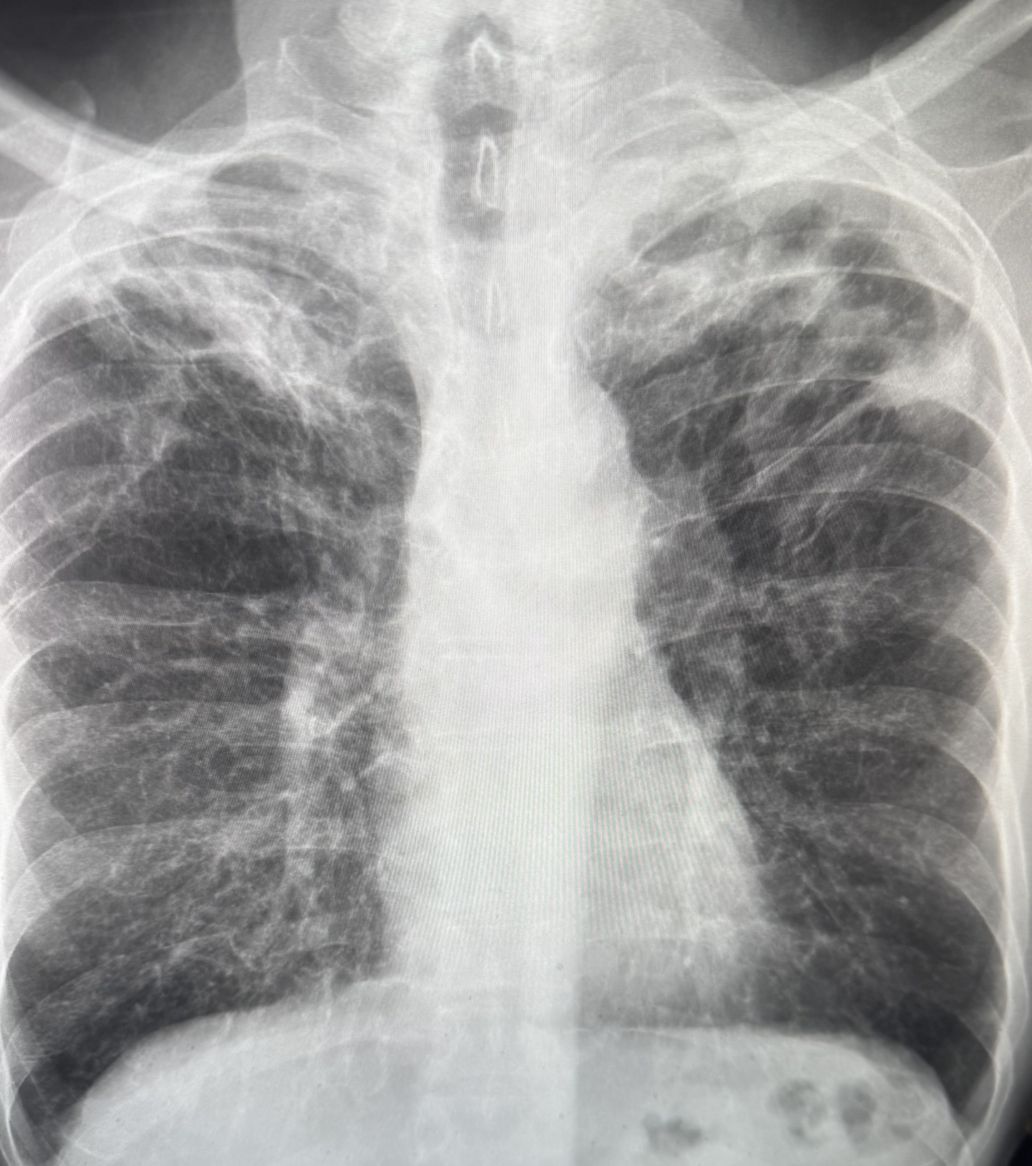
Anterior-posterior chest X-Ray revealing bi-apical lung fibrosis with decrease in the bi-apical opacities and cystic lucencies as compared to before treatment
